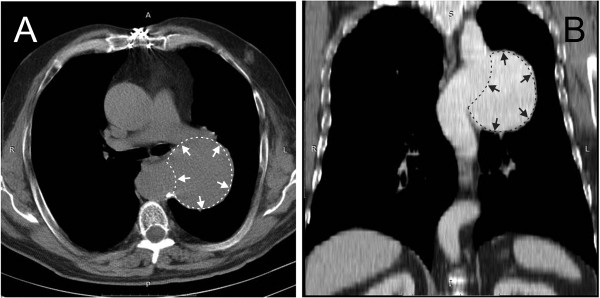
Contrast-enhanced computed tomography (CT) scan: transversal section (A) frontal plane bi-dimensional reconstruction (B). Image shows a 91×80×71mm aneurysm of the descending aorta.
